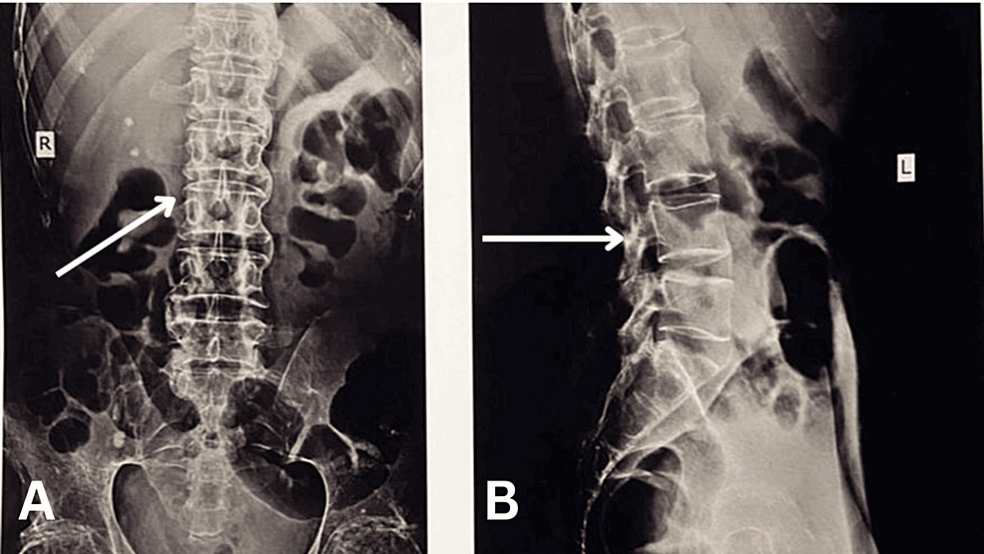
Lumbosacral spine X-ray. (A) Anteroposterior view showing bamboo spine appearance. (B) Lateral view of the lumbosacral spine where curvature has disappeared.
